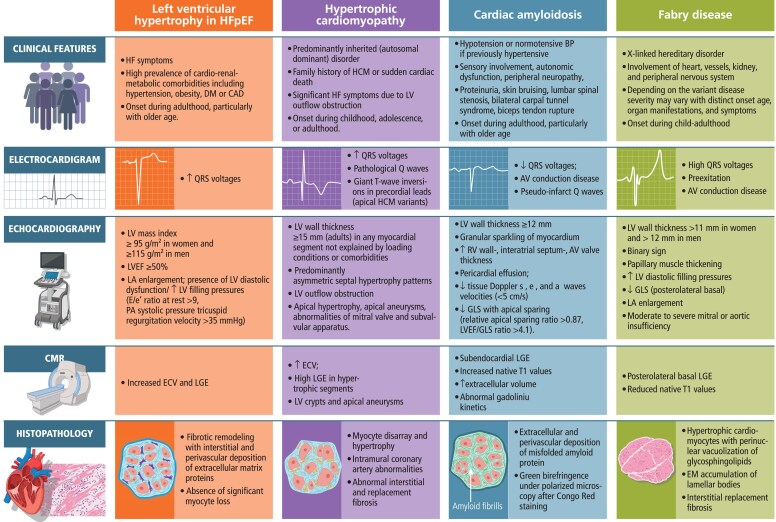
Differential characteristics and diagnosis of HFpEF with left ventricular hypertrophy and other cardiomyopathies with hypertrophic phenotypes. Left ventricular mass index is determined based LV mass and body surface area. AV, atrioventricular; BP, blood pressure; CAD, coronary artery disease; CMR, cardiovascular magnetic resonance; DM, diabetes mellitus; E/e′, early filling velocity on transmitral Doppler/early relaxation; ECV, extracellular volume; EM, extracellular matrix; GLS, global longitudinal strain; HCM, hypertrophic cardiomyopathy; HFpEF, heart failure with preserved ejection fraction; LA, left atrium; LV, left ventricle; LGE, late gadolinium enhancement; LVH, left ventricular hypertrophy; PA, pulmonary artery
